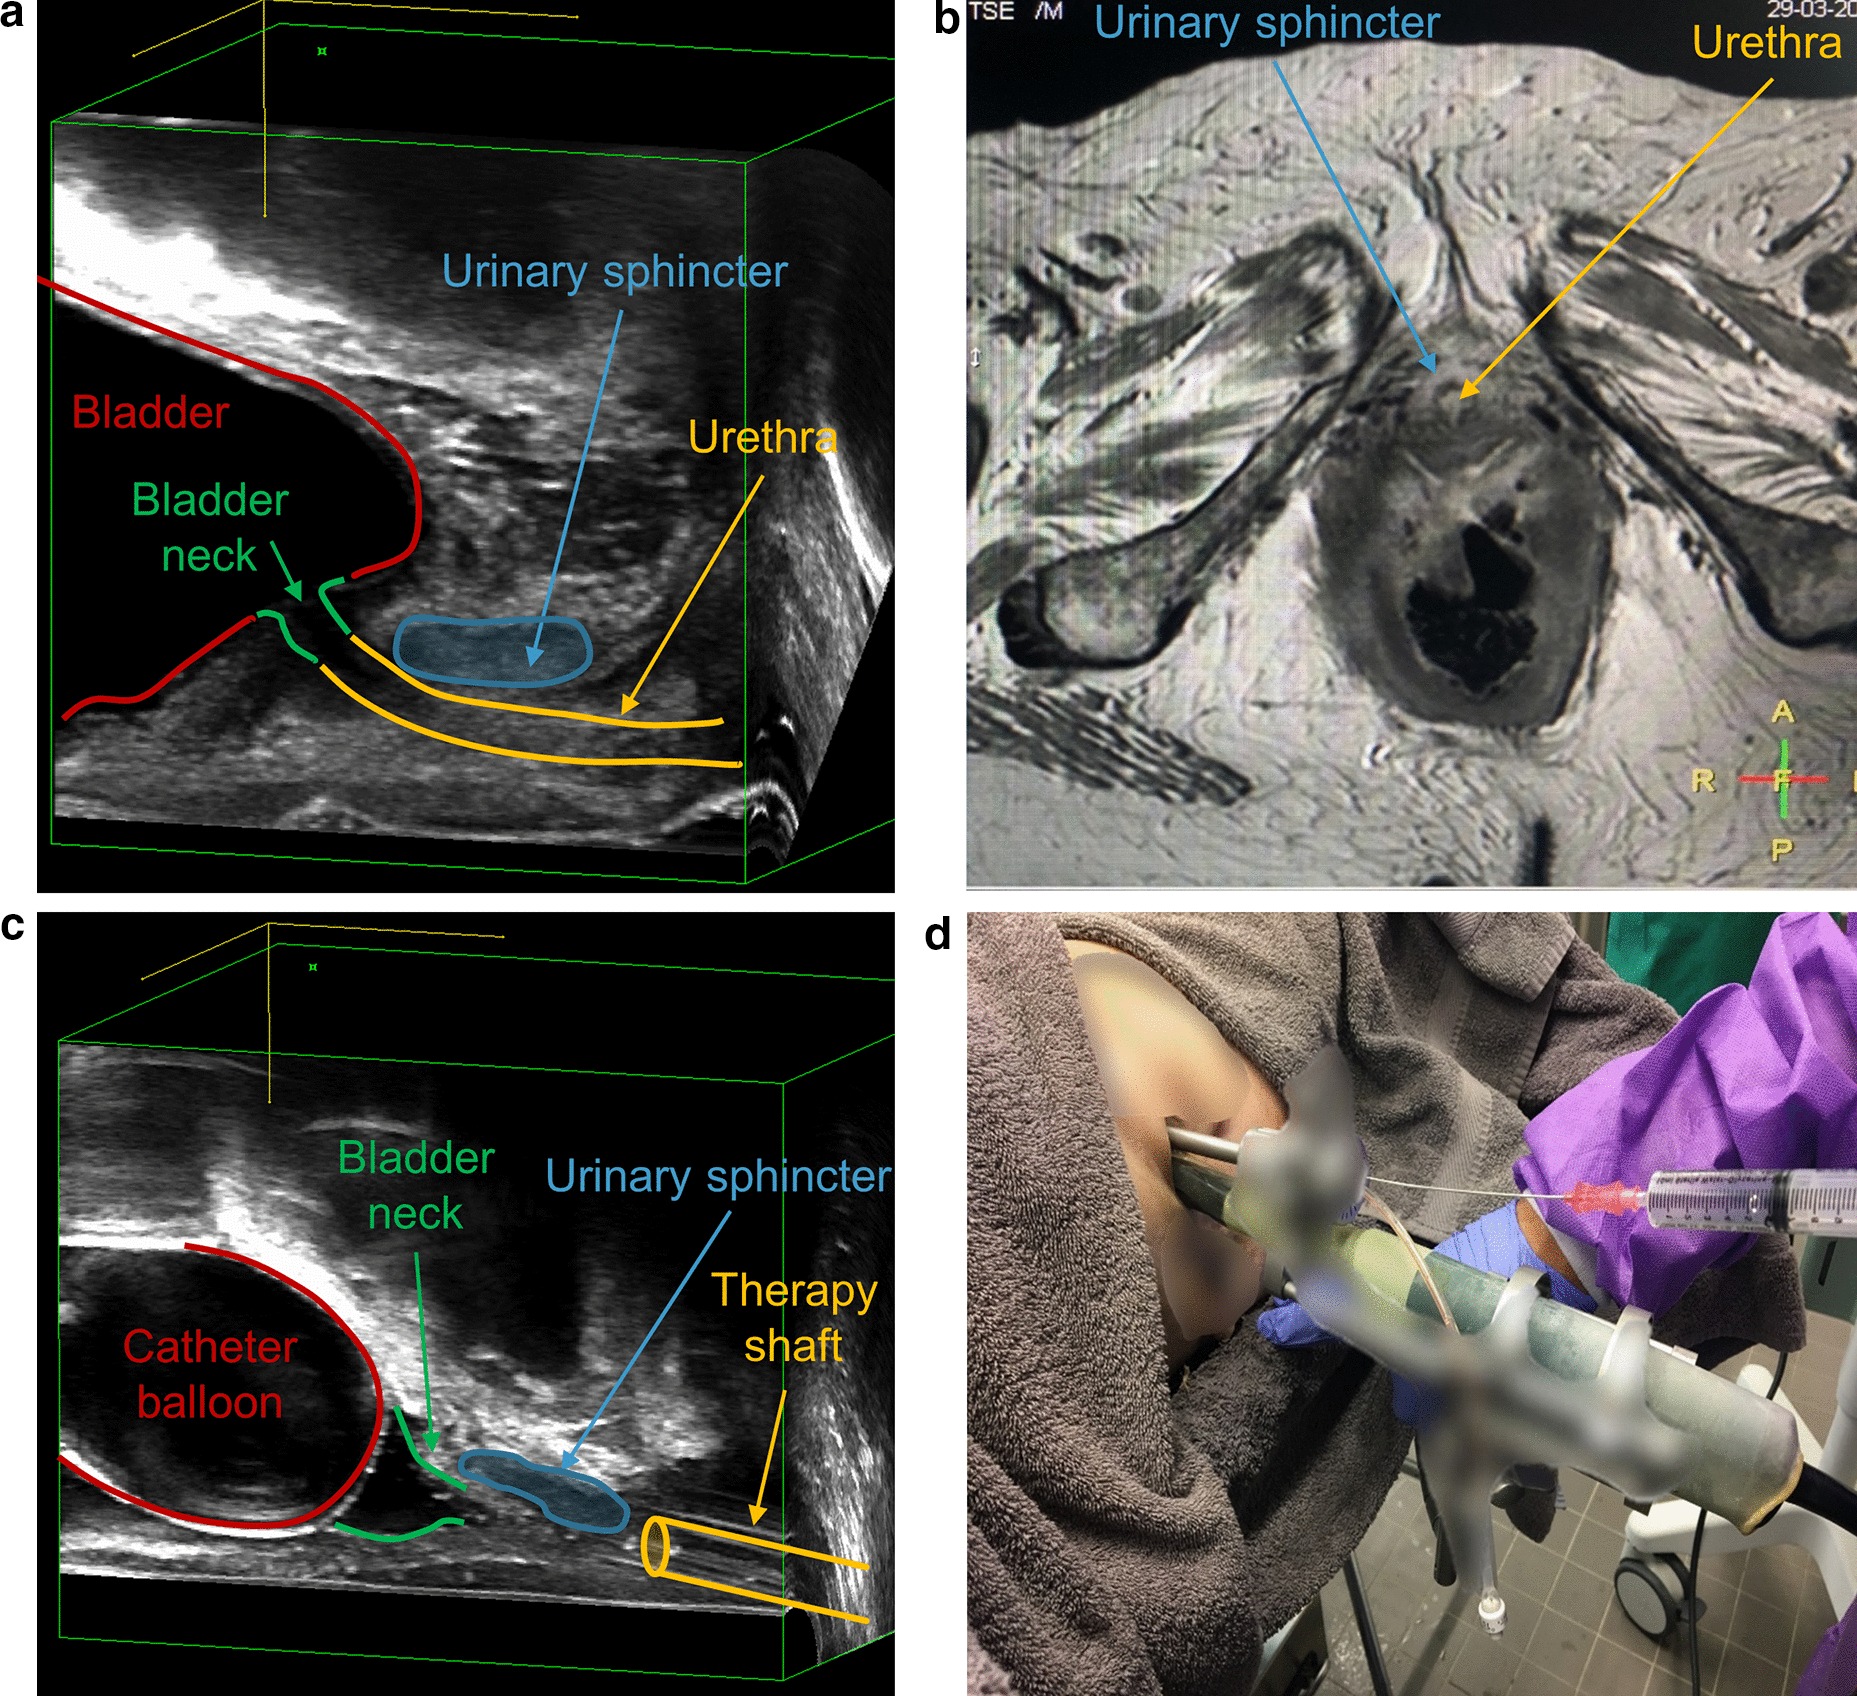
a Anatomy of the lower female pelvis in 3D US with transversal view and b MRI T2 sequence in axial view. c Setting before transurethral injection of nano-iron particles. Extracorporeal setup, where vaginal US probe and urethral therapy shaft are linked together. d Needle puncture and injection are performed through the therapy shaft
